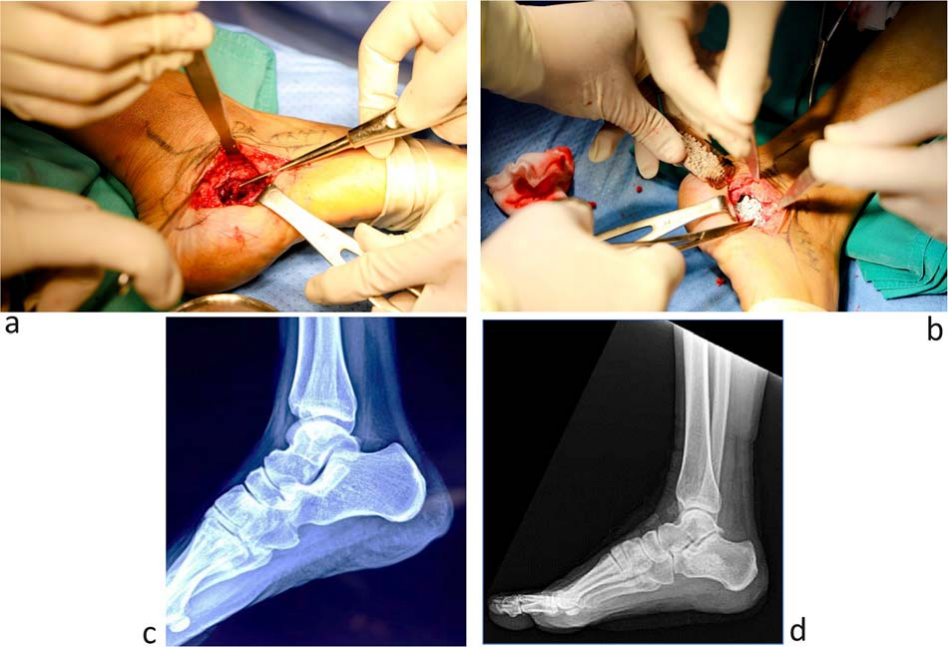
Images taken during surgery (a and b). A rectangular piece of cortical bone was dissected, revealing an area of tumor with sides 2.5 cm × 1.5 cm × 1.5 cm including yellow gray, poorly demarcated, soft neoplasm surrounded by red spongy bone tissue. A curette was used to remove the tumor and the surrounding irregular tissues until a solid dense cortical bone was reached (a). A unit of synthetic bone graft was well-compacted in the socket (b). Radiography of the right calcaneus before and one month after surgery (c and d, respectively) showed that the bone edge was not deformed (d).
